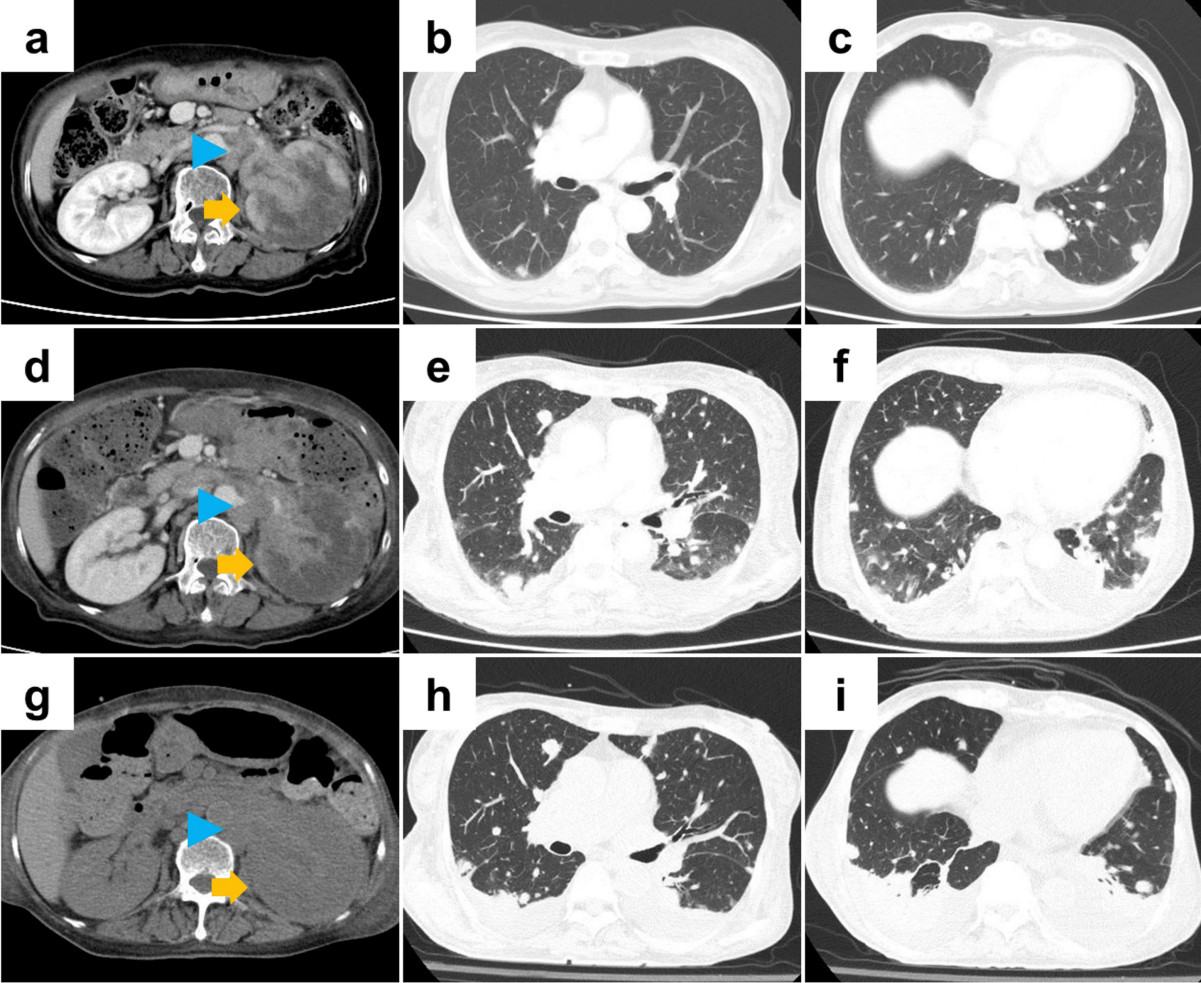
Computed tomography (CT) findings in Case 3 (a-c) Baseline contrast-enhanced CT at the time of diagnosis of metastatic disease. (a) Left renal pelvic mass infiltrating the renal parenchyma with suspected perirenal fat invasion, accompanied by multiple renal hilar lymph node metastases. (b, c) Multiple bilateral pulmonary metastases. (d-f) CT performed 8 days after renal biopsy. (d) Interval enlargement of renal hilar lymph node metastases. (e, f) Increase in both the number and size of multiple lung metastases, with new bilateral pleural effusions. (g-i) CT obtained 16 days after initiation of first-line enfortumab vedotin plus pembrolizumab, showing progressive disease. (g) Further enlargement of the left renal pelvic primary tumor and renal hilar lymph node metastases. (h, i) Continued increase in pulmonary metastases and worsening bilateral pleural effusions. Yellow arrows indicate the left renal pelvic primary tumor. Blue arrowheads indicate renal hilar lymph node metastases.
